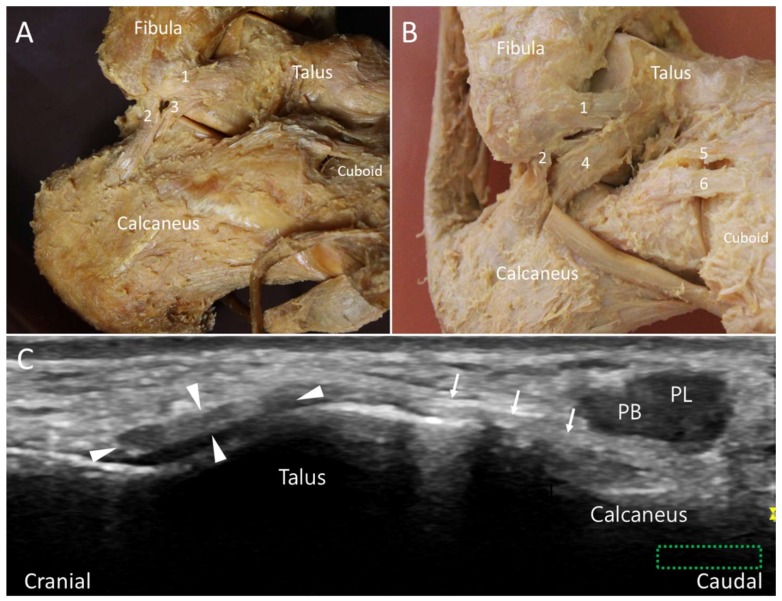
Type I (A) and type II (B) lateral talocalcaneal ligament in the cadaveric models. Ultrasound imaging shows the ligament (arrows) in its long axis (C). The colored dashed rectangle is in accordance with the transducer positions in Figure 2A. 1: Anterior talofibular ligament (arrowheads); 2: calcaneofibular ligament; 3: type I lateral talocalcaneal ligament; 4: type II lateral talocalcaneal ligament; 5: calcaneonavicular component of the bifurcate ligament; 6: calcaneocuboid component of the bifurcate ligament. PB: peroneus brevis tendon; PL: peroneus longus tendon.
